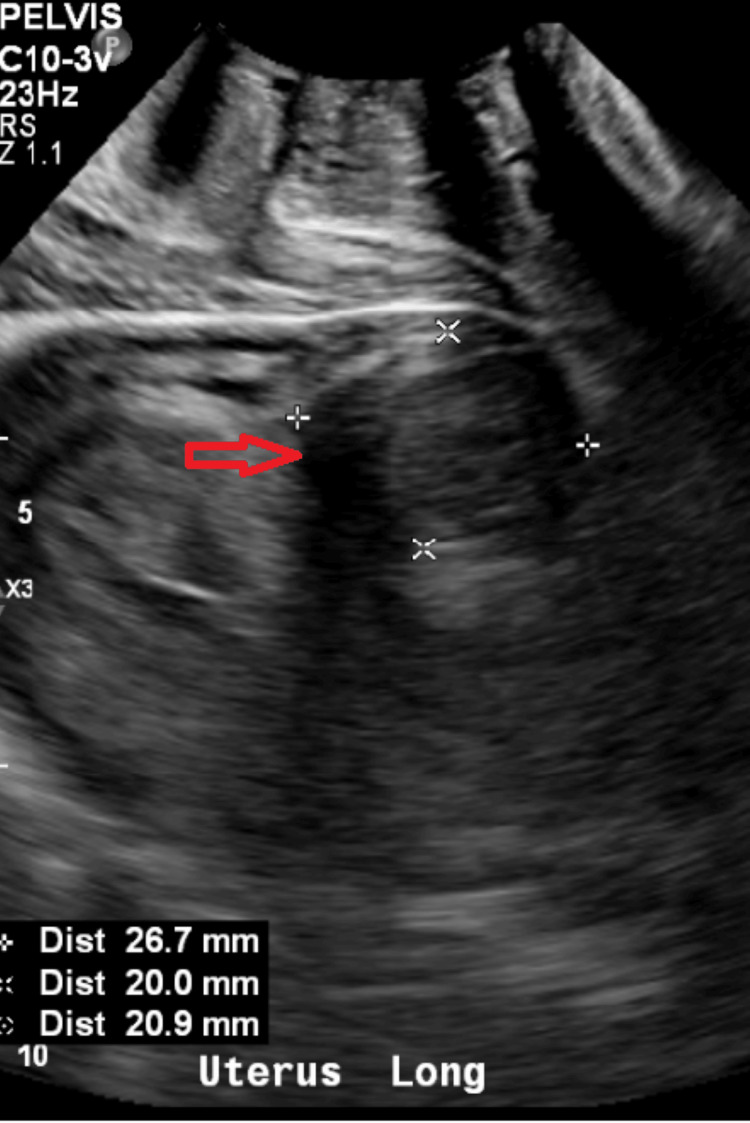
Pelvic ultrasound scan demonstrating uterus with a bulky fibroid measuring 27 × 20 × 21 mm (red arrow).
